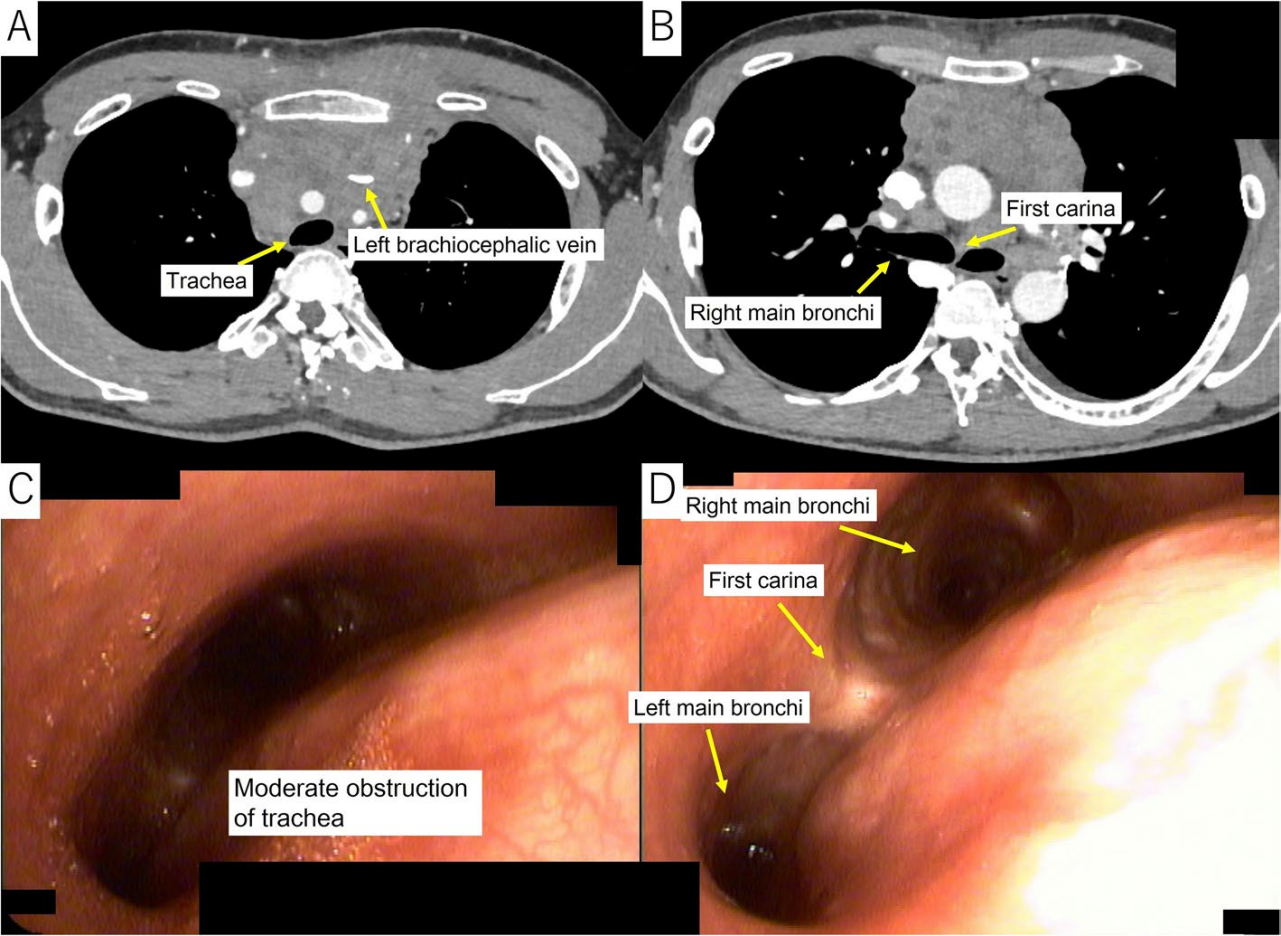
Contrast-enhanced computed tomography (CT) image showing a massive anterior mediastinal tumor with complete obstruction of the left brachiocephalic vein and moderate obstruction of the trachea (A), but no significant stenosis of the right main bronchus (B). Bronchoscopy showing moderate stenosis of the trachea (C) but no significant stenosis of either the left or right main bronchus (D), consistent with the CT images (A and B)
